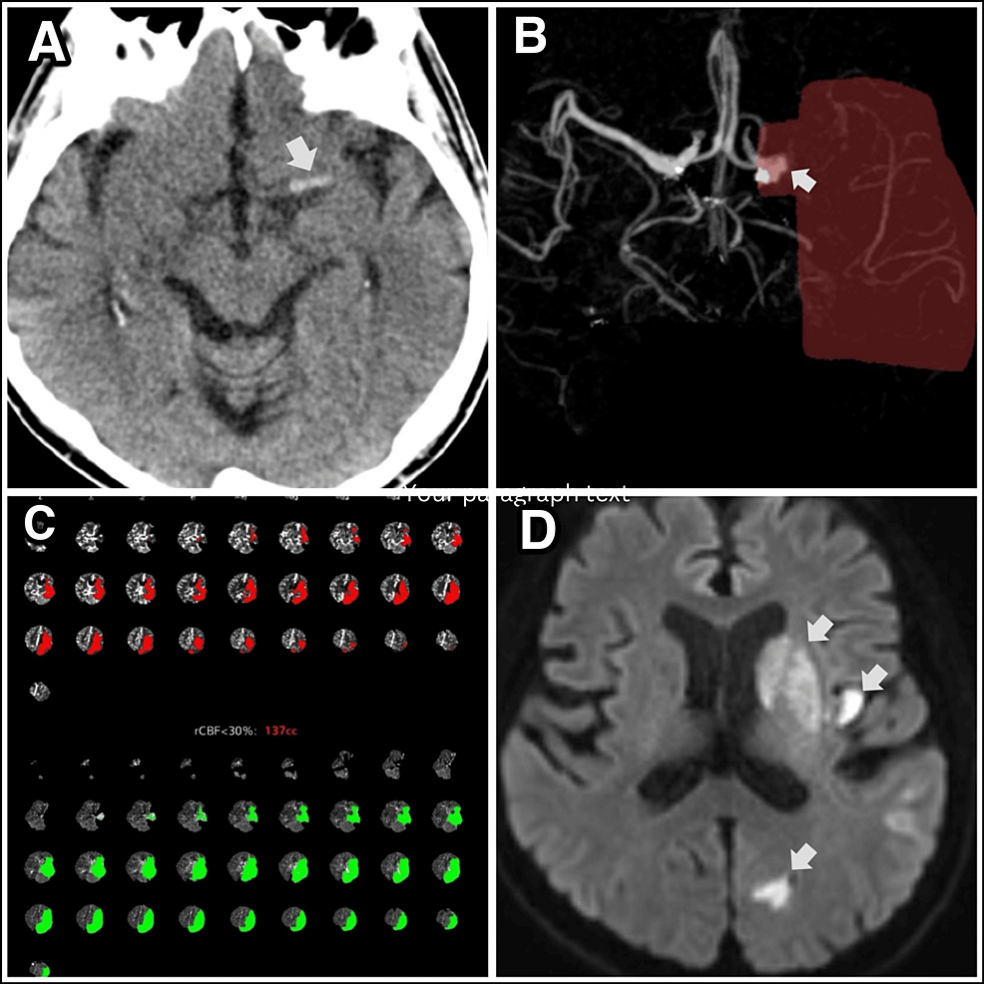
Imaging of a patient with an acute ischemic embolic stroke A) CT scan of the head shows a left hyperdense left middle cerebral artery (MCA) sign (arrow), B) Rapid ® artificial intelligence software showing the left MCA M1 clot (arrow), C) VizAi ® showing  Mismatch ratio 1.8 ( Tmax > 6sec- 251 mL, CBF <30%-137 mL). D) MRI of the brain DWI sequence showing restricted diffusion involving the left basal ganglia (top arrow), portions of the insular cortex on the left (middle arrow), and ischemia in the region of the left occipital lobe (bottom arrow). The patient had a fetal origin of the posterior cerebral artery( PCA); therefore, an infarction in the occipital lobe.
